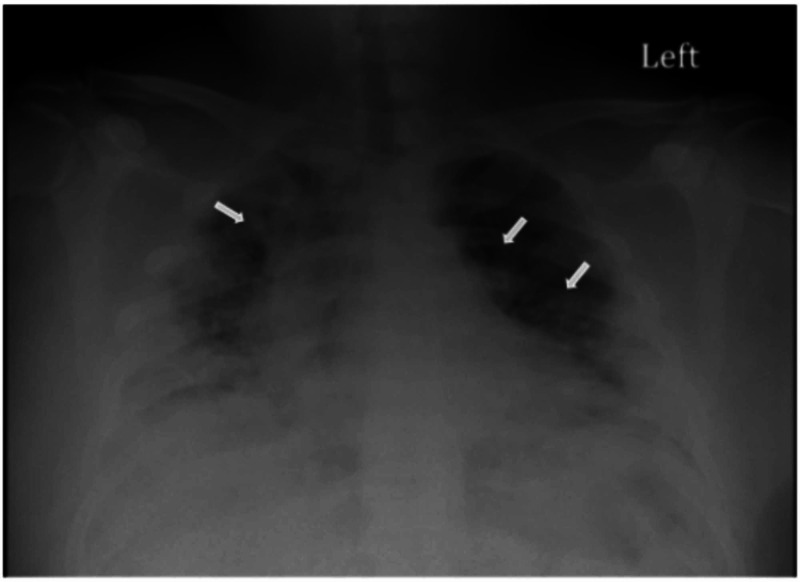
Chest X-ray taken during the patients' initial hospital visit, showing diffuse bilateral pulmonary disease (white arrows)
